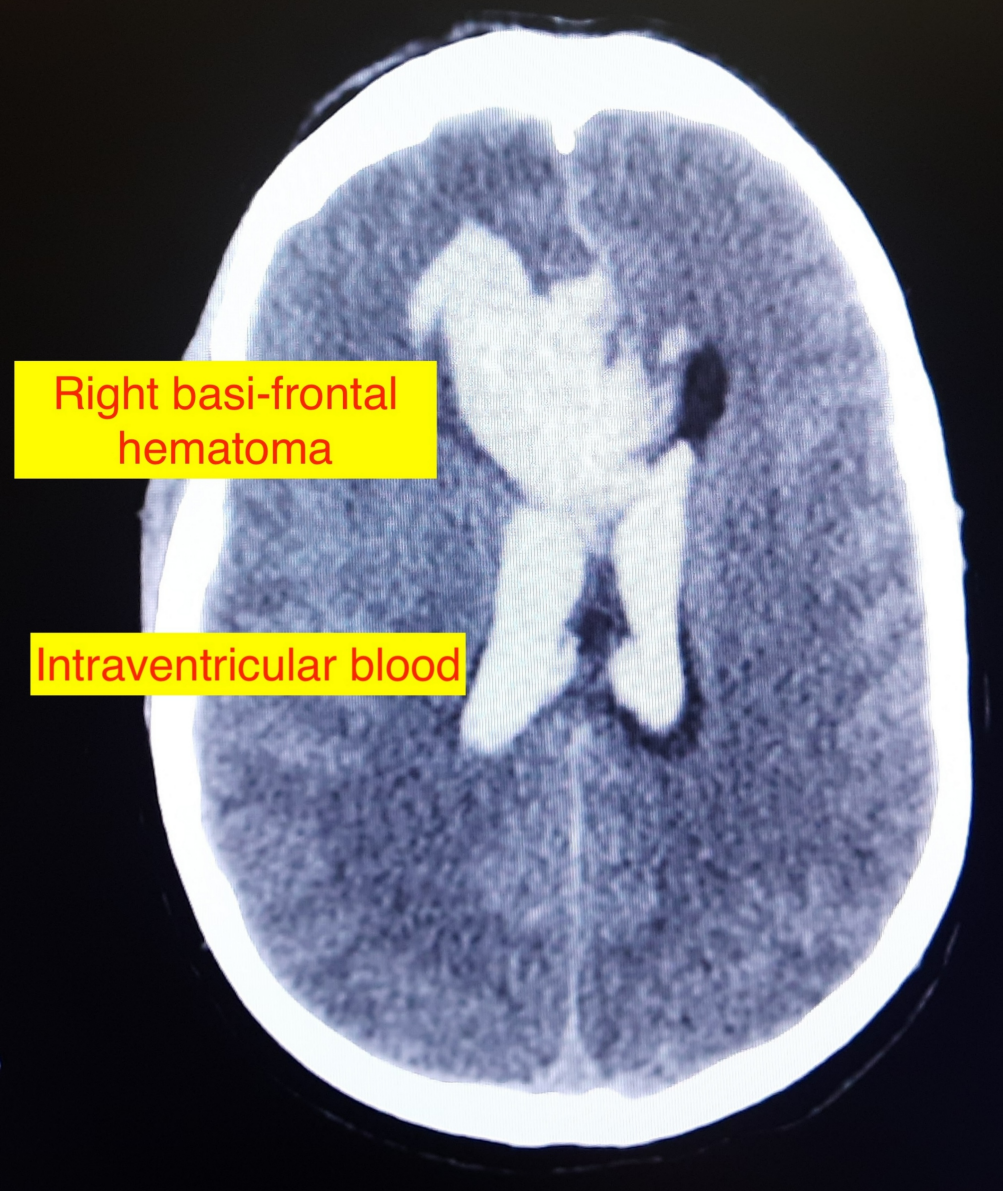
Non-contrast computed tomography of the head showing right basi-frontal hematoma with intraventricular extension.
